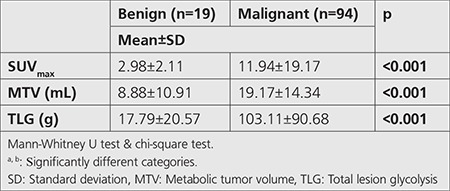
Positron emission tomography/computed tomography findings of benign and malignant solitary pulmonary nodules
